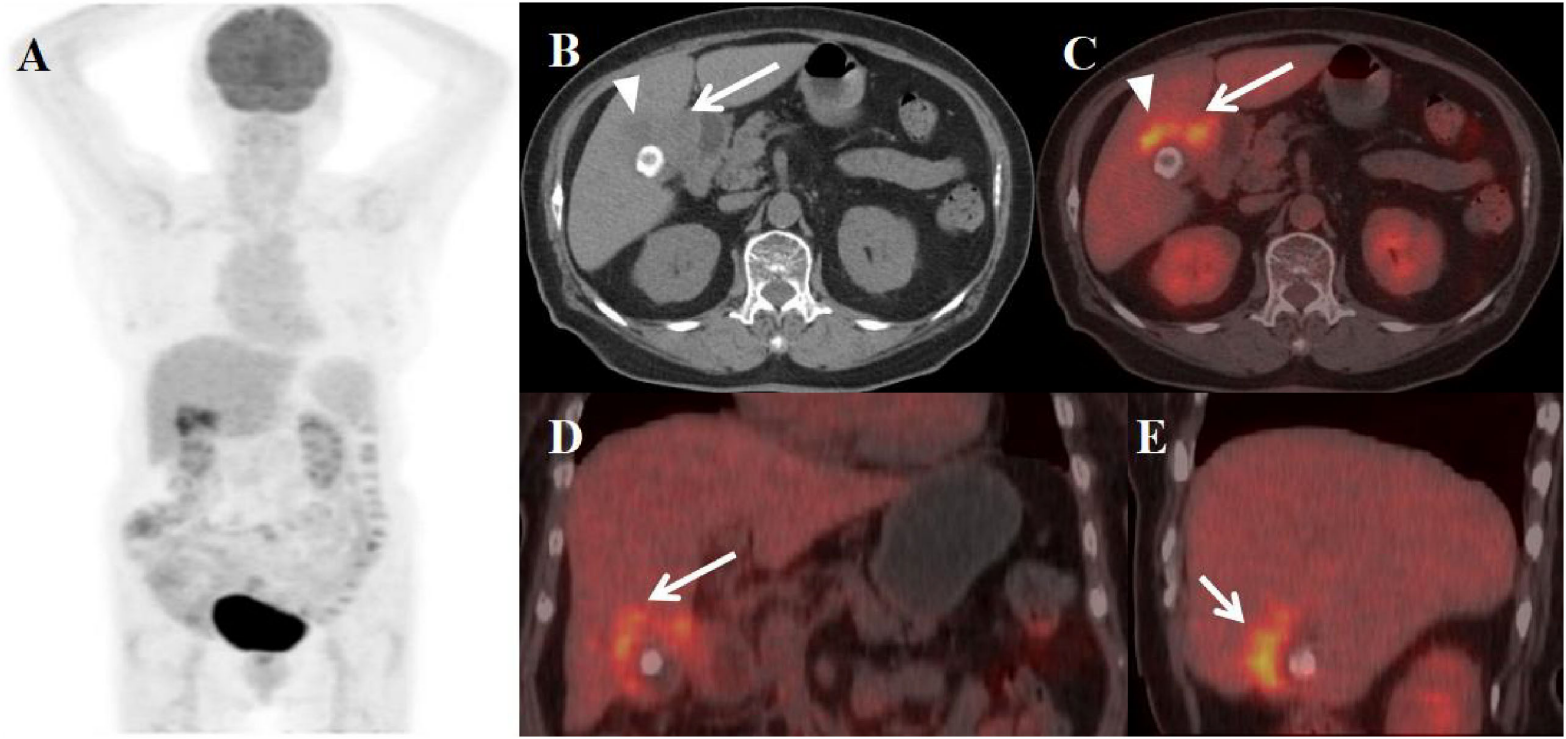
Female, 84 years old. (A) (whole body MIP), (B, C) (Axial CT and fused axial PET-CT), (D) (Fused coronal PET-CT), and (E) (Fused sagittal PET-CT). 18F-FDG PET/CT Imaging Findings: The gallbladder demonstrates diffuse wall thickening (maximum 1.9 cm) with luminal narrowing (A, arrow ↑), while maintaining mucosal integrity. The hepatobiliary interface appears indistinct, with a 2.3 cm mildly hypodense hepatic lesion noted adjacent to the gallbladder (B, arrow ▲). The thickened gallbladder wall shows duodenal bulb adhesion, with both the mural thickening and adjacent hepatic lesion exhibiting moderate FDG avidity (SUVmax 6.5). Associated cholelithiasis is present.
